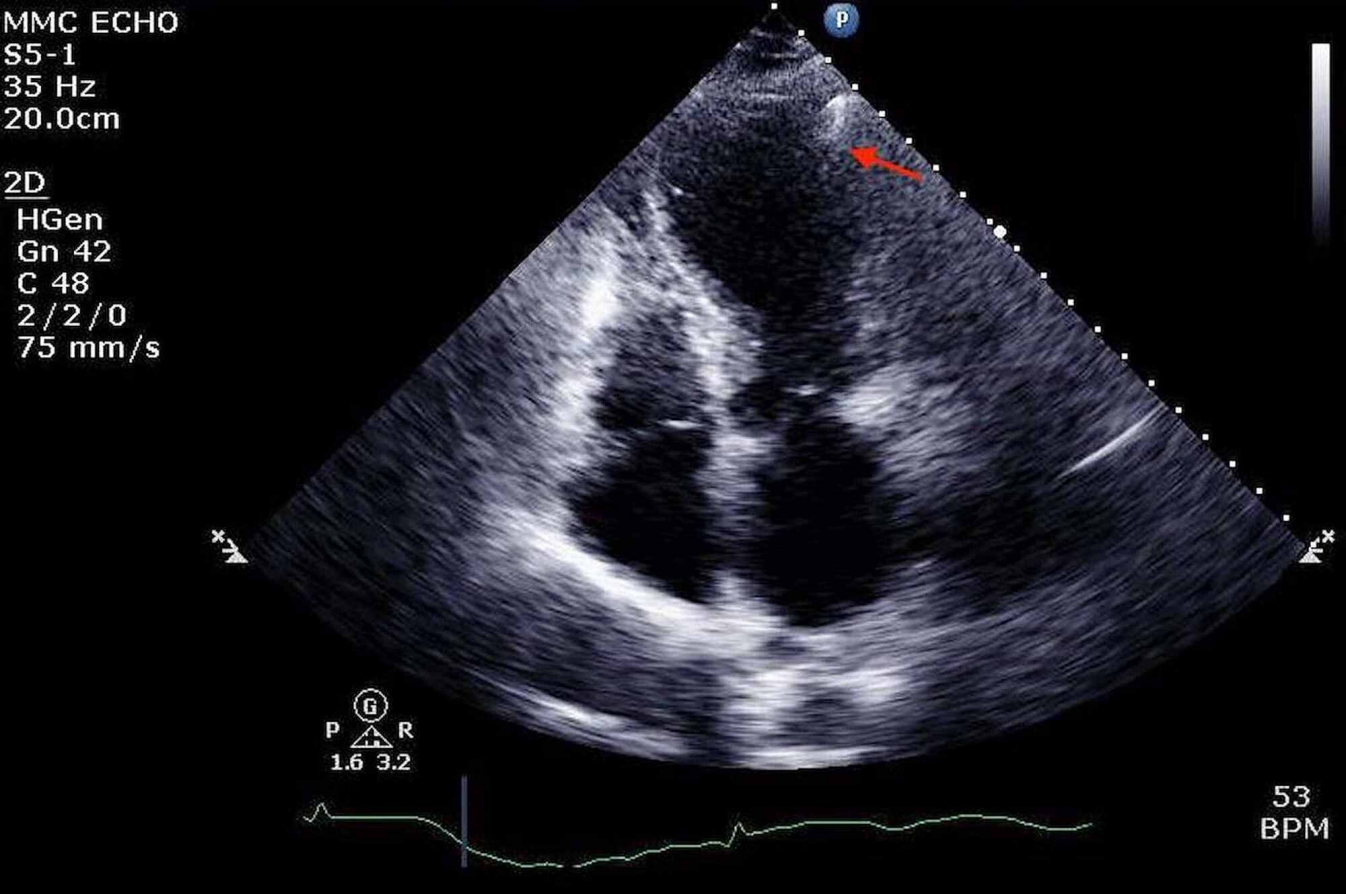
Transthoracic echocardiogram showing apical ballooning (red arrow). Apical four-chamber view showing systolic apical ballooning consistent with TC. TC: Takotsubo cardiomyopathy.
